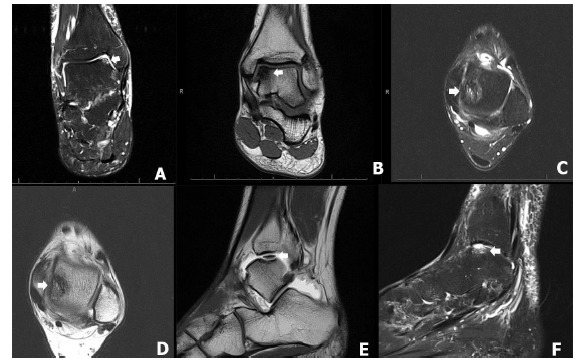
The MRI demonstrates the osteochondral lesions of the talus (OLT). The coronal plane of the MRI demonstrates anteromedial lesions of OLT, Hepple stage 1 and 2A in figure 2A, and 2B (arrow), respectively. The axial MRI demonstrates mid-medial lesions of the OLT, Hepple stage 2A in figure 2C and 2D. The sagittal MRI demonstrates non-displaced mid-medial lesion of OLT, Hepple stage 3, in figure 2E (arrow) and displaced mid-lateral lesion of the OLT, Hepple stage 4, in (Fig. 2F) (arrow).
